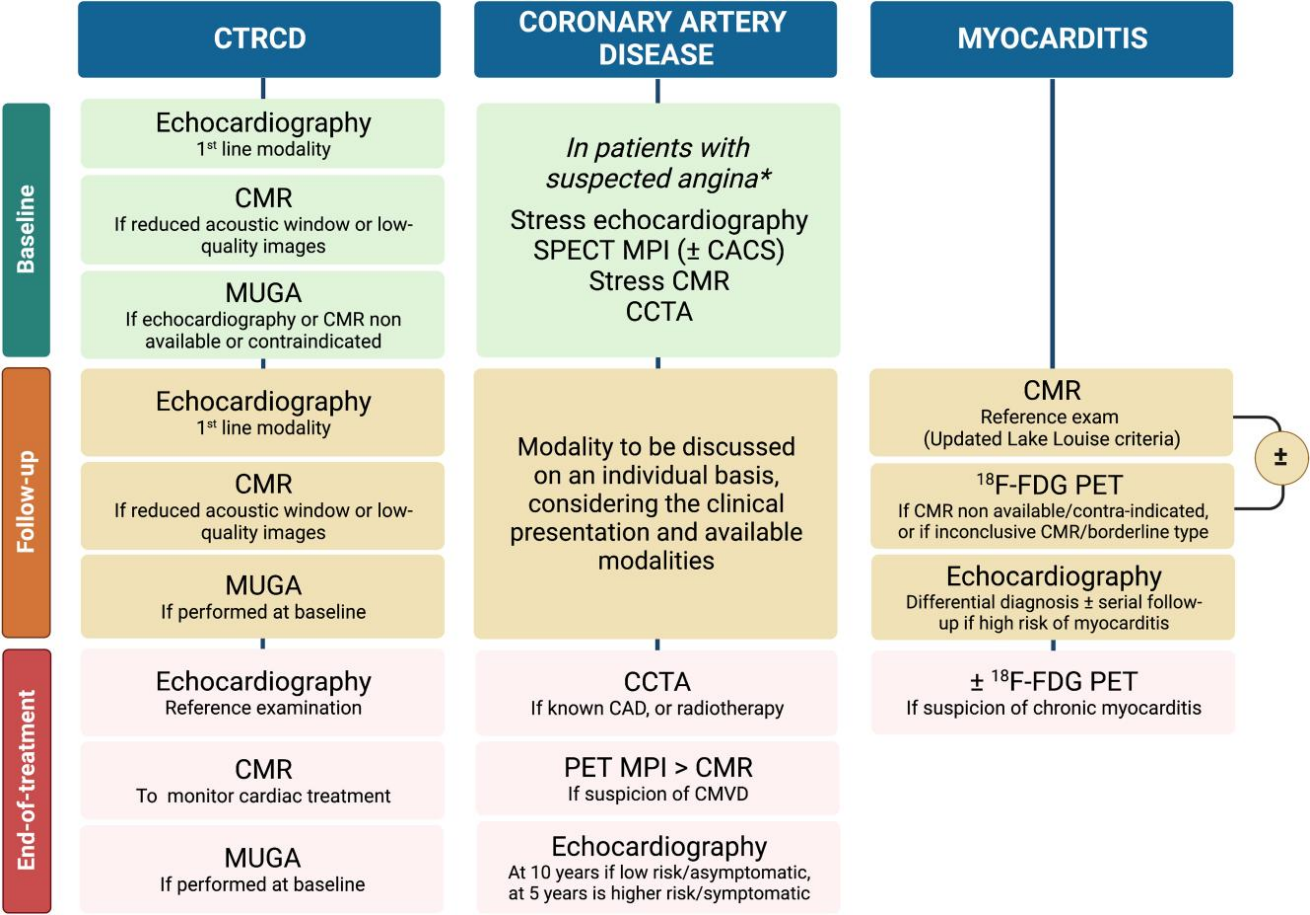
Algorithm proposal for non-invasive imaging in patients undergoing anticancer treatment. Abbreviations: ^18^F-FDG, fluor-18-radiolabelled fluorodeoxyglucose; CACS, coronary artery calcium score; CAD, coronary artery disease; CCTA, coronary computed tomography angiography; CMR, cardiac magnetic resonance; CTRCD, cancer-treatment-related cardiac dysfunction; LVEF, left ventricular ejection fraction; SNMMI, Society of Nuclear Medicine and Molecular Imaging; MPI, myocardial perfusion imaging; MUGA, multigated acquisition; PET, positron emission tomography; SPECT, single-photon emission computed tomography. *The choice of imaging modality should be based on symptoms, known CAD, pretest probability, local availability and expertise, and patient characteristics.
